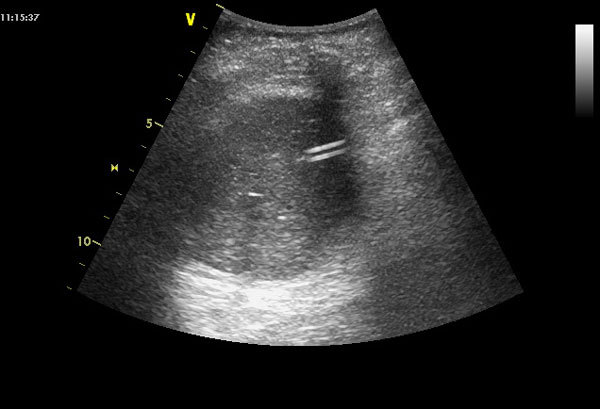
Assessment of intragastric position of a small bore enteral feeding tube by ultrasonography [31]. The probe is placed in the middle epigastric area and oriented toward the left upper abdominal quadrant to visualize the gastric area. The small bore feeding tube appears as two parallel hyperechogenic lines.
